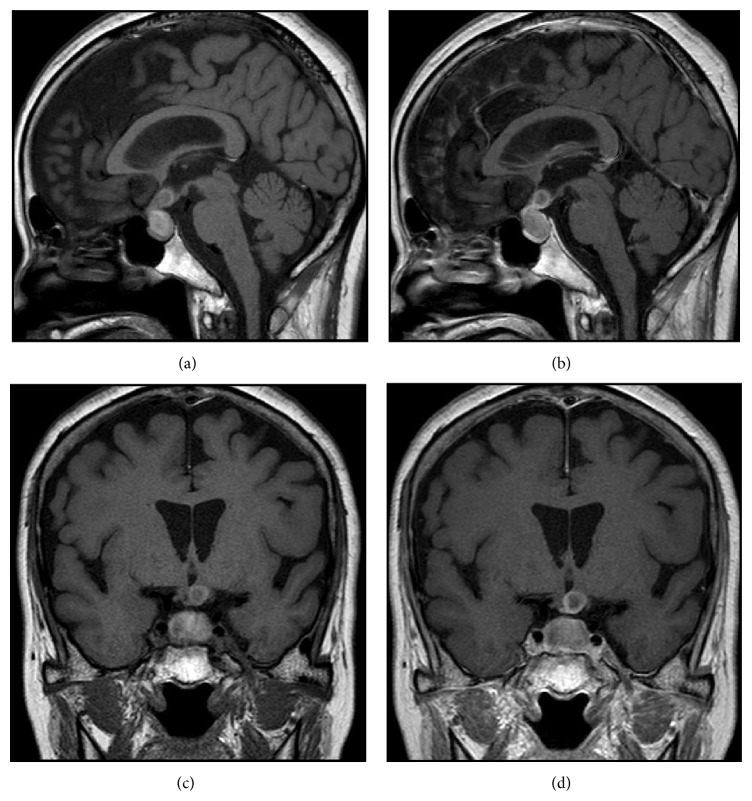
Brain MRI (T1-weighted images). Sagittal views of (a) plain and (b) Gd-enhanced images and coronal views of (c) plain and (d) Gd-enhanced images are shown.
